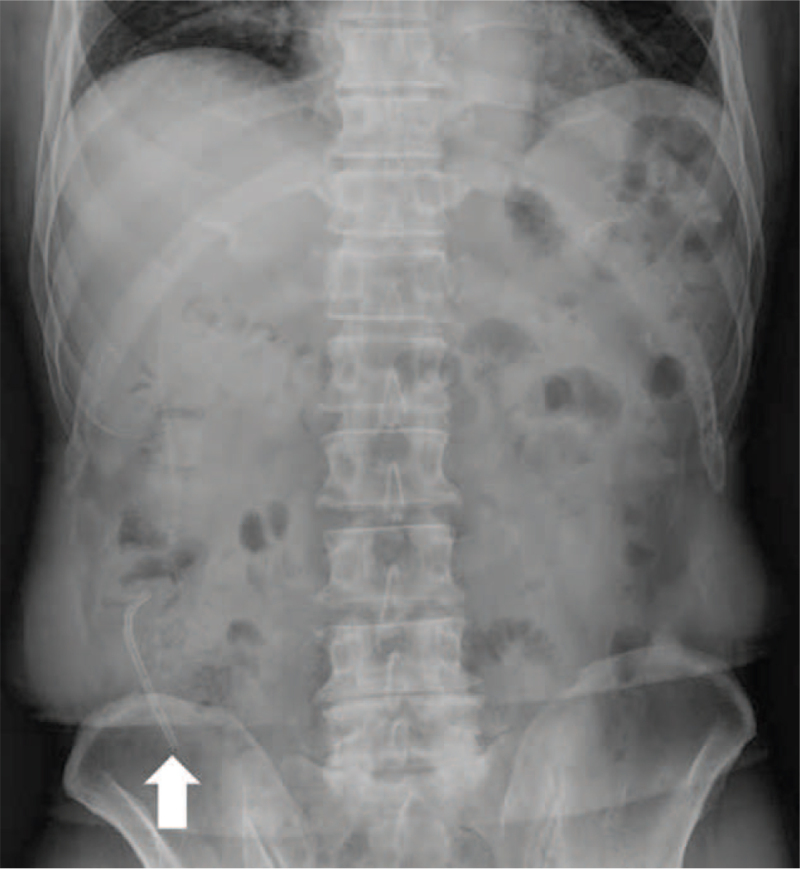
On abdominal radiography, the migrated endobiliary stent (arrow) is noted in the right lower quadrant area.
